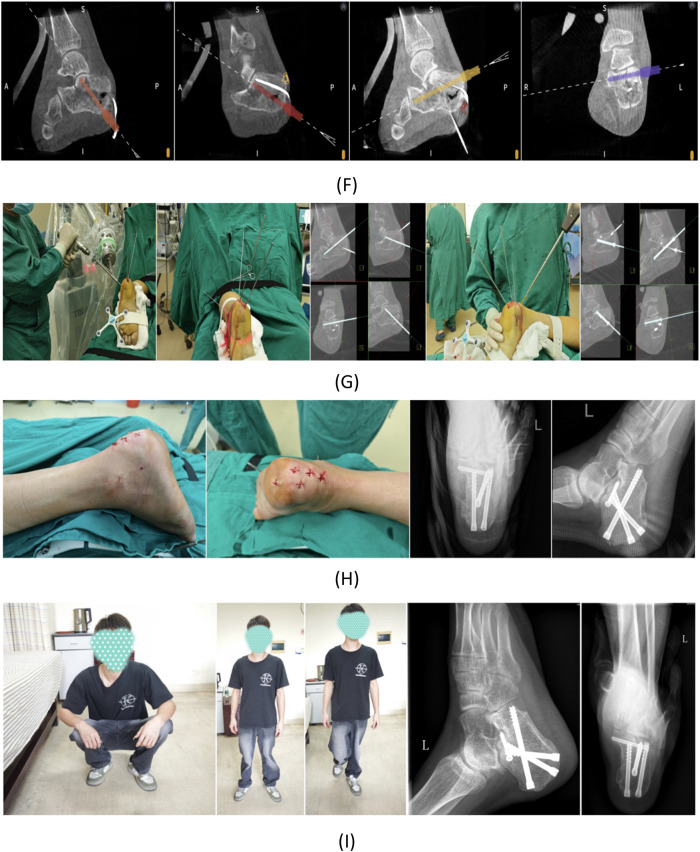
Case presentation: A 24-year-old male patient sustained a left calcaneal fracture due to a fall from height. (A) Preoperative CT scan of the calcaneus. (B) Routine disinfection and draping were performed. The left foot was securely fixed using a foot holder with a tracker attached. (C) Sterile working environments were established for both the C-arm image intensifier and the robotic arm. (D) After acquiring 3D intraoperative imaging data and uploading them to the robotic surgical planning platform, trajectories were planned for K-wires used in reduction. Two 2.5 mm K-wires were robotically inserted, followed by manual insertion of a 4.0 mm K-wire for calcaneal traction. After all K-wire placements, closed manual reduction was performed by the surgeon with assistant support. (E) Post-reduction, a 2.5 mm K-wire was temporarily placed for fracture stabilization, with reduction quality verified by 3D imaging. (F) Four screws were planned for definitive fracture fixation. (G) Robot-assisted insertion of four guide pins was performed, with subsequent 3D imaging confirming their positions. Satisfactory guide pin placement was followed by screw insertion and positional verification. (H) After confirming proper screw placement, the incision was closed, and postoperative calcaneal radiographs were obtained. (I) At 3-month follow-up, complete fracture healing was observed without screw loosening or displacement. The patient demonstrated excellent functional recovery with pain-free ambulation and normal gait.
